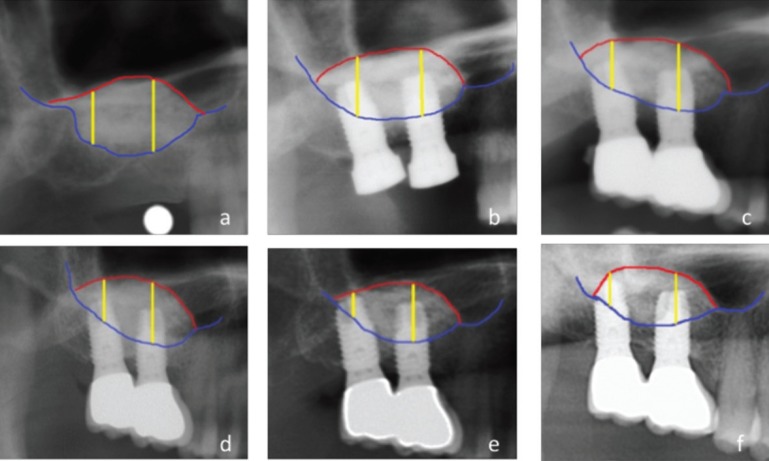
Panoramic X-ray measurements made to evaluate bone graft height at the different study timepoints. a) Sinus lift; b) Implant placement; c) Prosthetic loading; d) 12 months after loading; e) 5 years after loading; f) Maximum follow-up, 9 years after loading.
